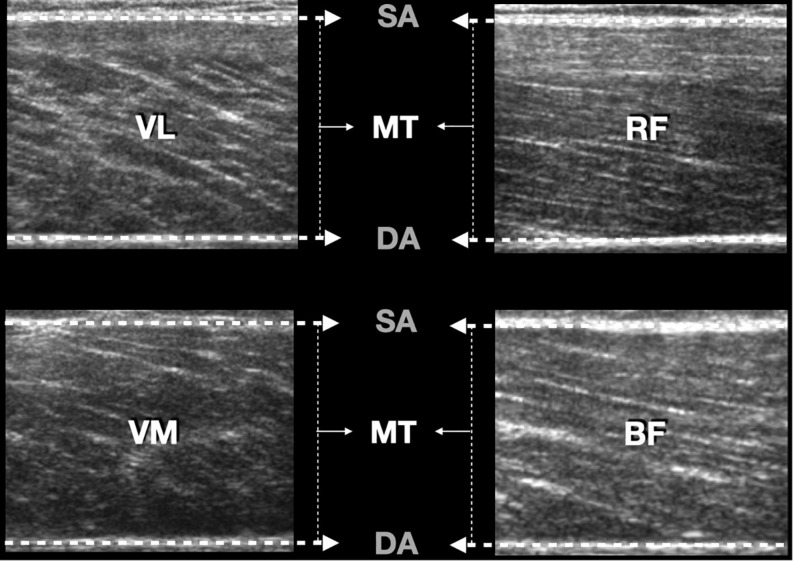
Example of ultrasound image showing the cross-cutting scans of the muscles. SA, superficial aponeurosis; DA, deep aponeurosis; MT, muscle thickness; VL, vastus lateralis; RF, rectus femoris; VM, vastus medialis; BF, biceps femoris.
